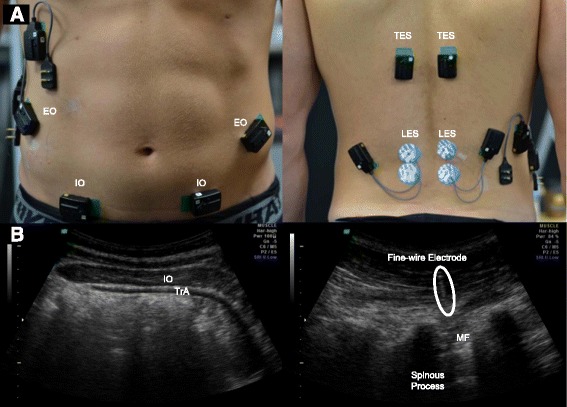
a Experimental setup for surface EMG: external oblique (EO), internal oblique (IO), thoracic erector spinae (TES) and lumbar erector spinae (LES). b Ultrasound images of the muscles of interest for indwelling EMG: internal oblique (IO), transversus abdominis (TrA), and deep multifidus (MF)
